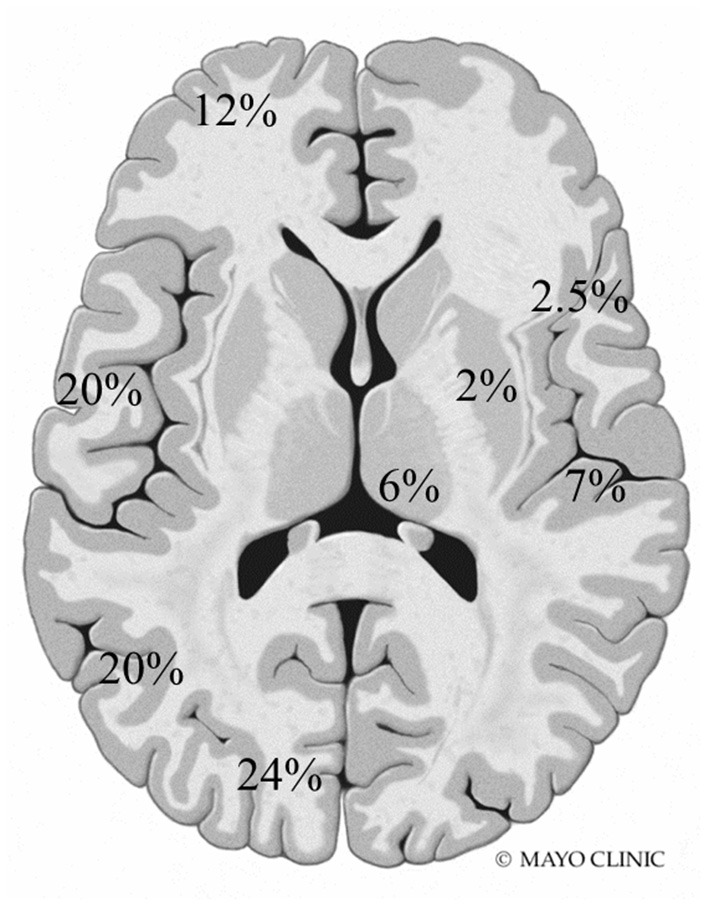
Frequency and location of MRI abnormalities. Right side: insula, perirolandic, basal ganglia, and thalamus. Left side: frontal, temporal, parietal, and occipital lobes (not shown: brainstem 0.5% and cerebellum 5%).
